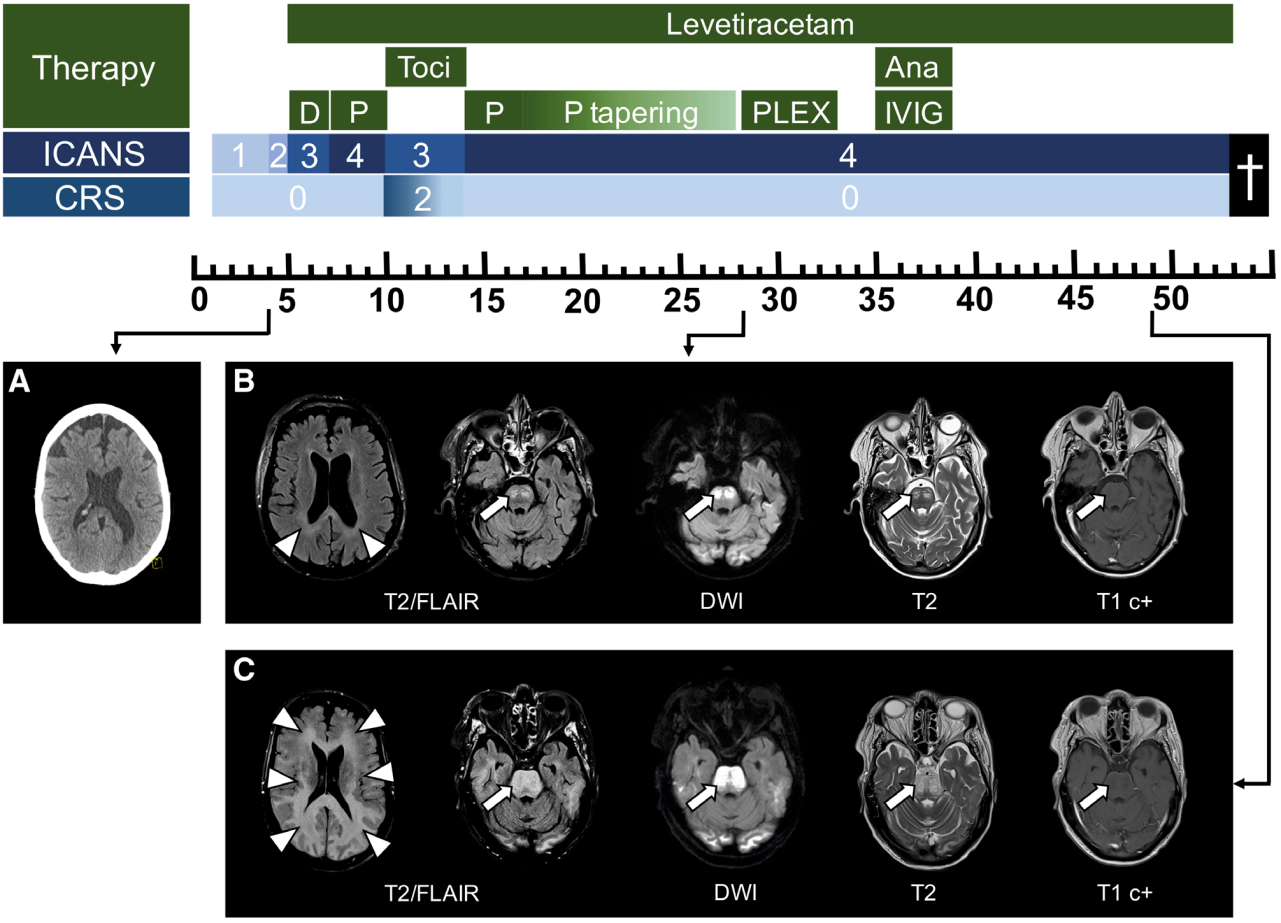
Timeline and management of neurotoxicity following CAR T-cell therapy. Upper part: Swimmer plot depicting the kinetics and management of neurotoxicity through 55 d after CAR T-cell infusion. The highest grade of neurotoxicity (ICANS; navy blue row), CRS (light blue row), and provided therapy (green rows) on each day are color-coded. Imaging studies are also indicated. (A), Computed tomography of the brain at onset of ICANS grade 2 showing unspecific frontal atrophy without intraaxial pathologies. (B), Axial T2/FLAIR- (left panel), DWI- (middle left panel), T2- (middle right panel), and T1-postcontrast (right panel; T1 c+) weighted MRI of the brain during ICANS grade 4 demonstrating symmetric white matter lesions extending along the posterior horn of the lateral ventricles (arrowheads) to the basilar part of the pons (arrows). Associated restricted diffusion indicating blood-brain-barrier dysfunction is present, whereas no contrast enhancement can be seen. (C), Axial T2/FLAIR- (left panel), DWI- (middle left panel), T2- (middle right panel), and T1-postcontrast (right panel; T1 c+) weighted MRI of the brain after several weeks of ICANS grade 4 displaying progression of the juxtaventricular supra (arrowheads) and pontine infratentorial white matter lesions (arrows). Severe pontine swelling with extensive diffusion restriction is shown, indicating profound edema of the brain stem due to blood-brain-barrier disruption. Ana = anakinra; CAR = chimeric antigen receptor; CRS = cytokine release syndrome; D = dexamethasone; DWI = diffusion-weighted imaging; FLAIR = fluid-attenuated inversion recovery; ICANS = immune effector cell–associated neurotoxicity syndrome; IVIG = intravenous immunoglobulins; MRI = magnetic resonance imaging; P = prednisolone; PLEX = plasma exchange; Toci = tocilizumab.
